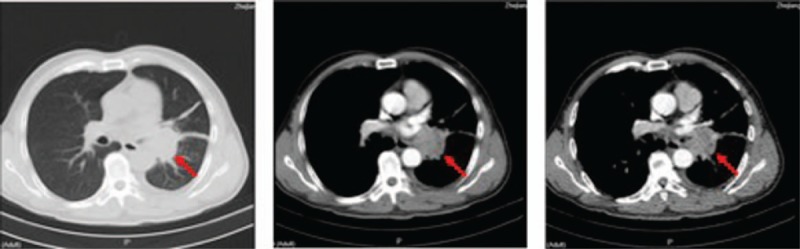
Enhanced CT scan confirmed the existence of neoplasm in the left lower lobe. Red arrows indicate the location of the tumor.
